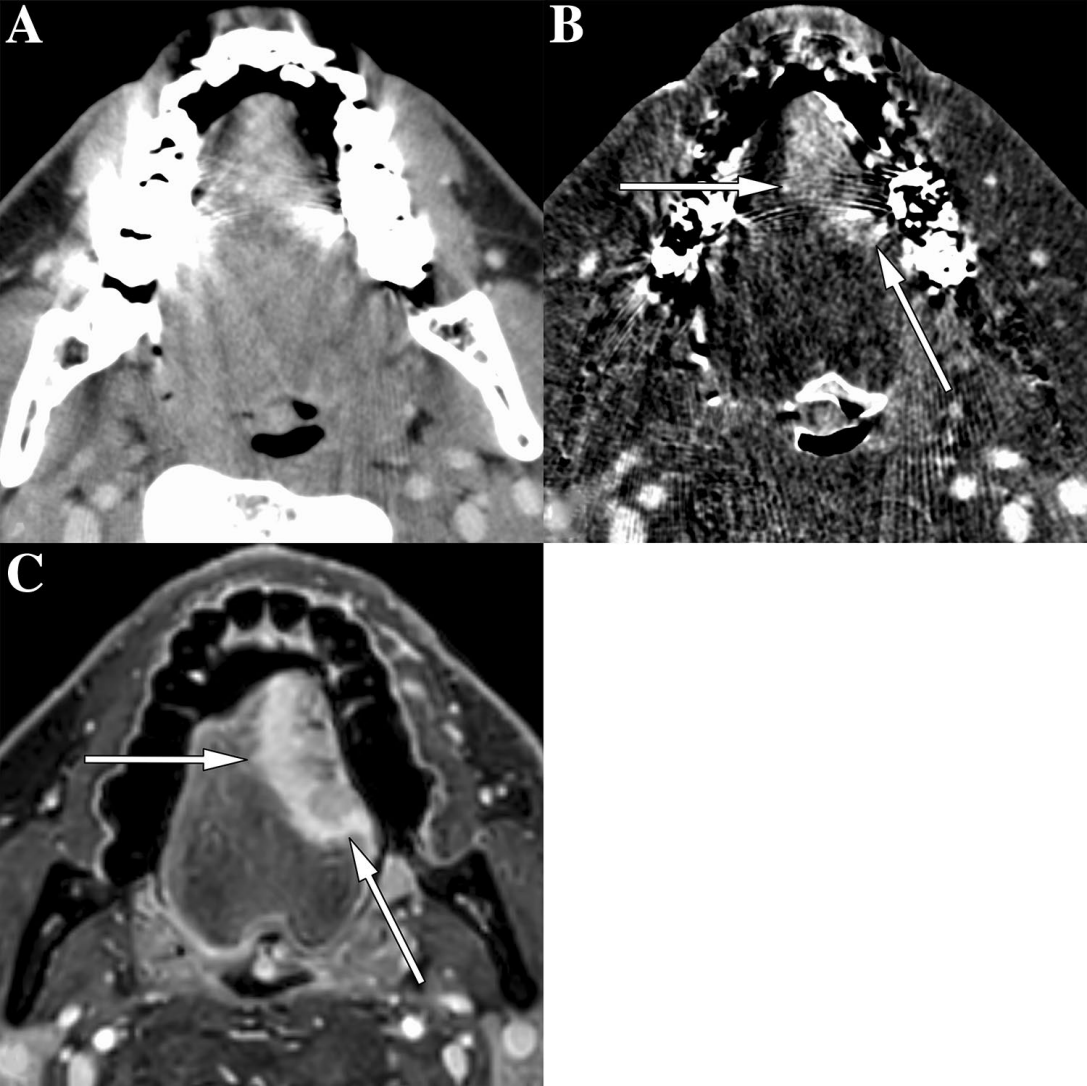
Images of a 43-year-old man with squamous cell carcinoma of the left border of the tongue. A On CT with single-energy projection-based metallic artifact reduction algorithm, tongue cancer was not detectable due to bilateral dental artifacts. B Subtraction iodine imaging (SII) is able to delineate the tumor mainly due to accentuation of iodine and increasing contrast between the tumor and tongue muscles. C On contrast-enhanced T1-weighted MR images with fat suppression, the tumor is detectable and measurable (white arrows in C) as well as with SII
